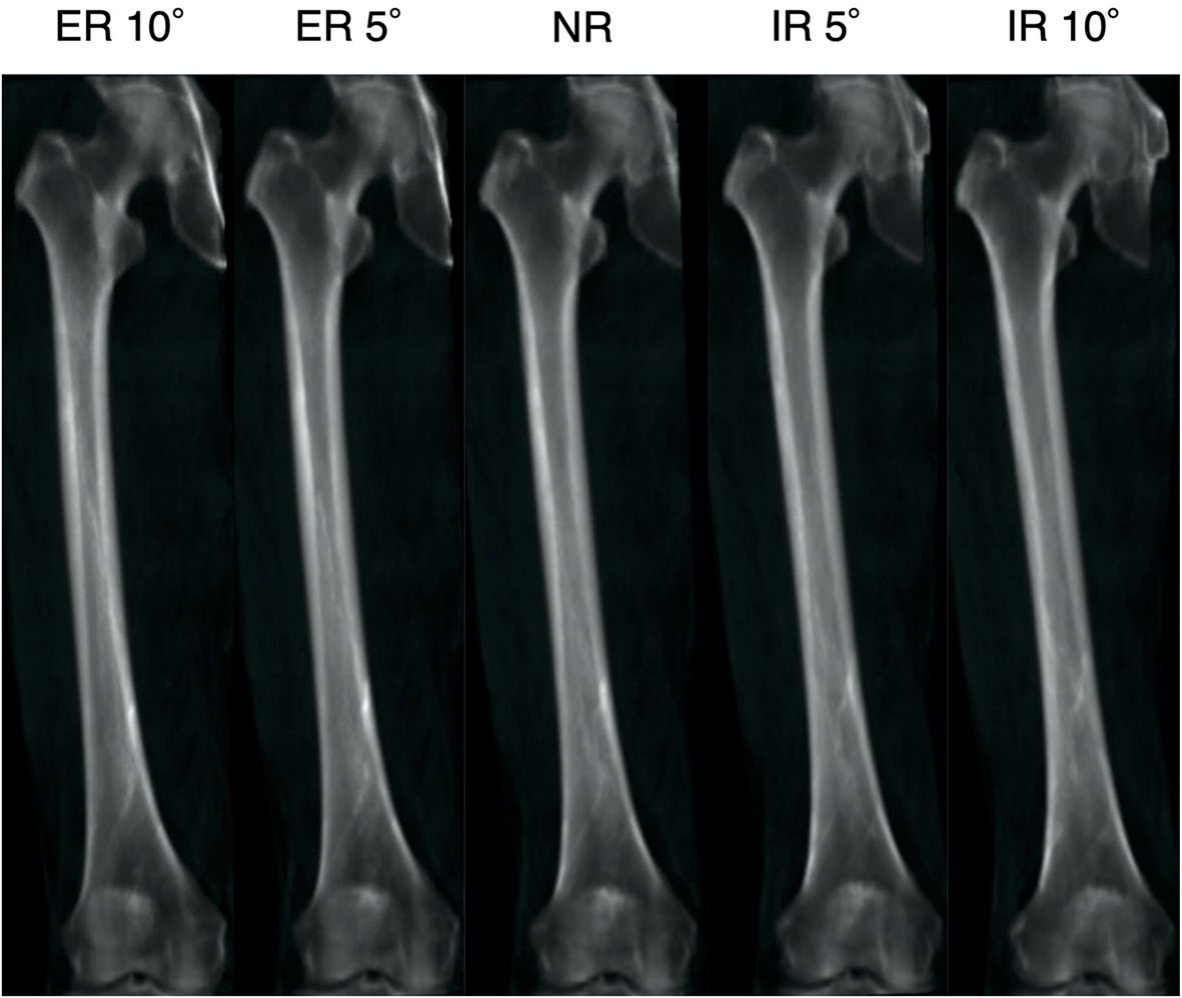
The digitally reconstructed radiography (DRR) images parallel to the SEA (NR), 5° and 10° relative to the SEA, respectively, were reconstructed. ER, external rotation; IR, internal rotation; NR, neutral rotation; SEA, surgical epicondylar axis
